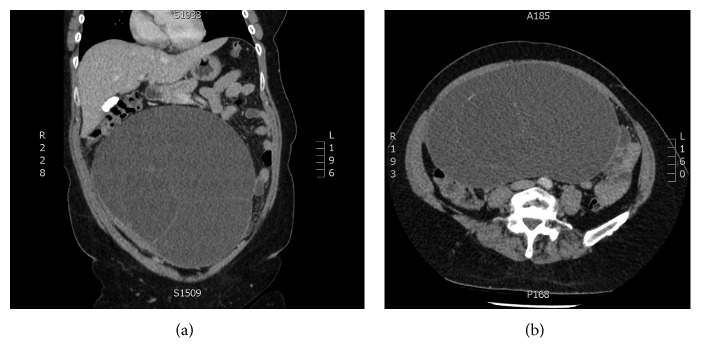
(a, b) CT abdomen showing large septated ovarian mass.
